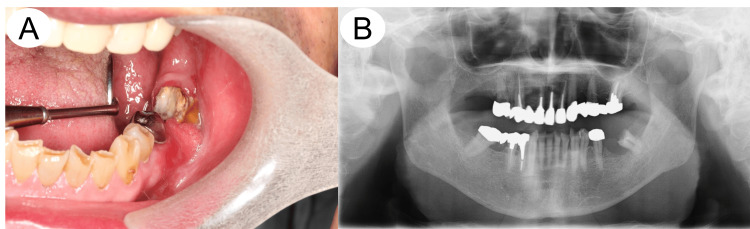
Clinical and panoramic findings at the first appearance A: Intraoral examination revealed a hemorrhagic mass measuring 20 × 15 mm in size, along with ulcer formation in the left buccal mandibular gingiva. B: Panoramic X-ray detected alveolar bone resorption with surrounding bone enhancing sclerotic changes in the left mandibular molar region.
